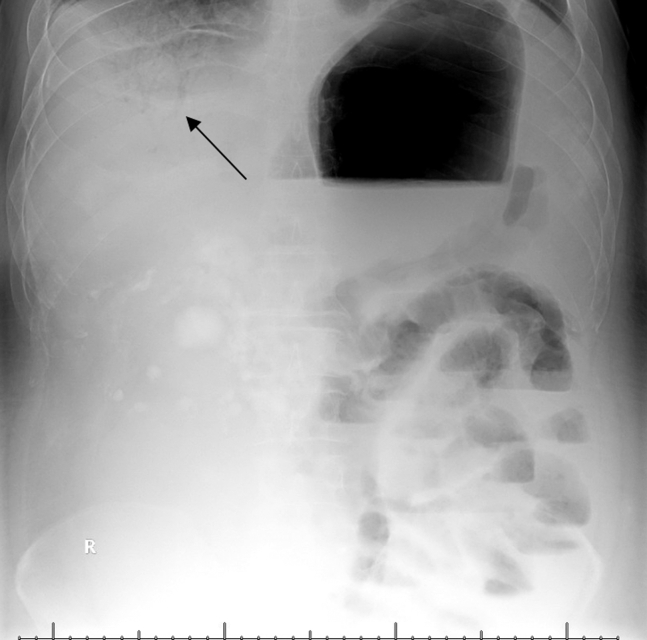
Portal venous gas on portable x-ray (black arrow).
